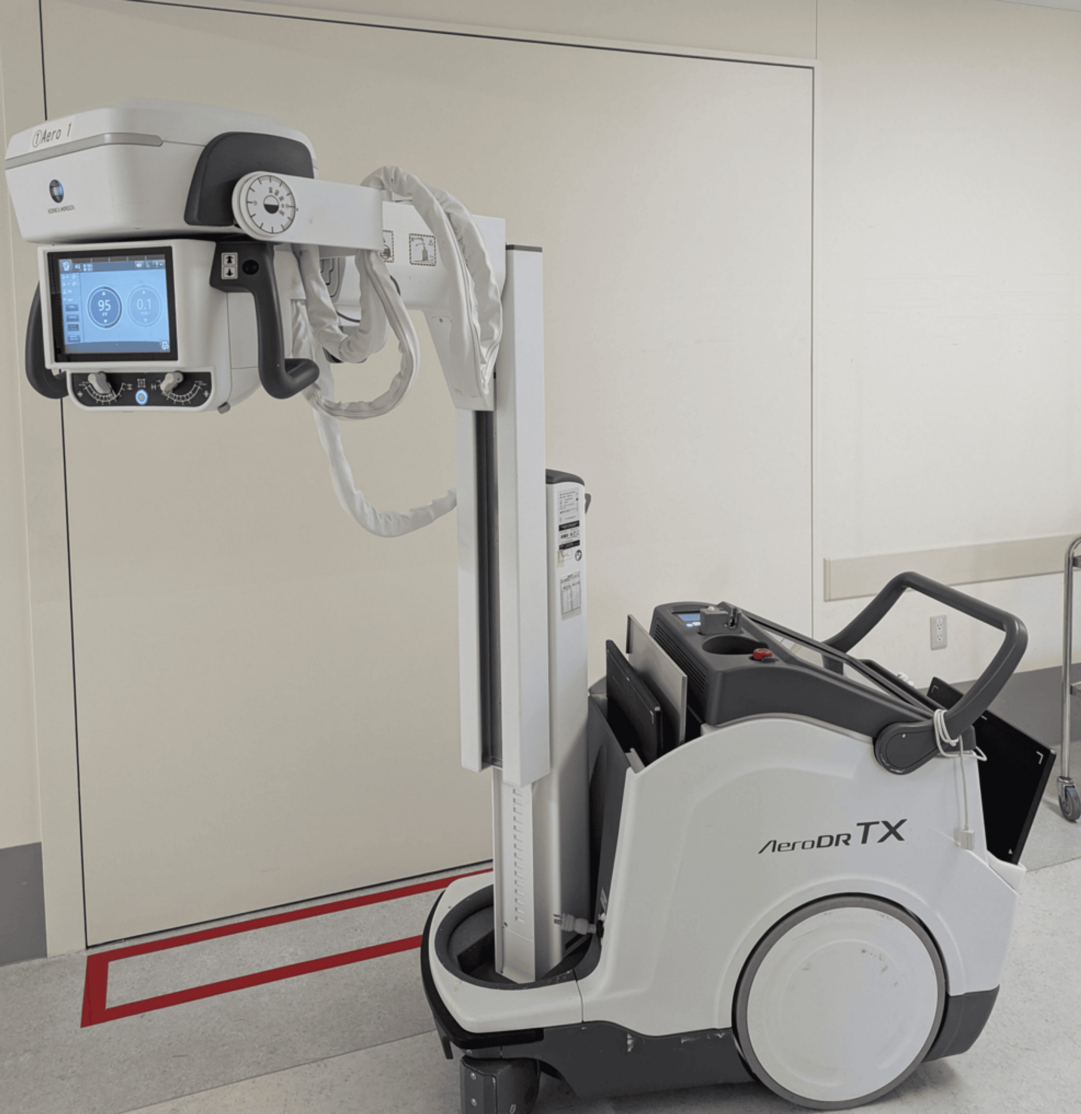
Photograph of the mobile dynamic digital radiography equipment (AeroDR Trauma & X-ray; Konica Minolta, Chiyoda City, Japan), which has a portable detector panel.
